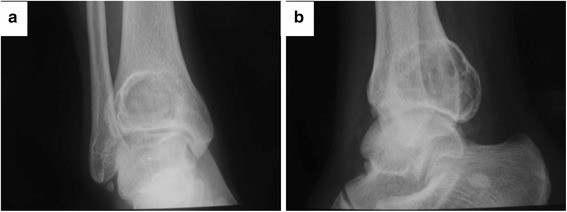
Pre-operative X-ray shows the lesion located in the distal of the right tibia. a Anteroposterior X-ray. b Lateral X-ray
